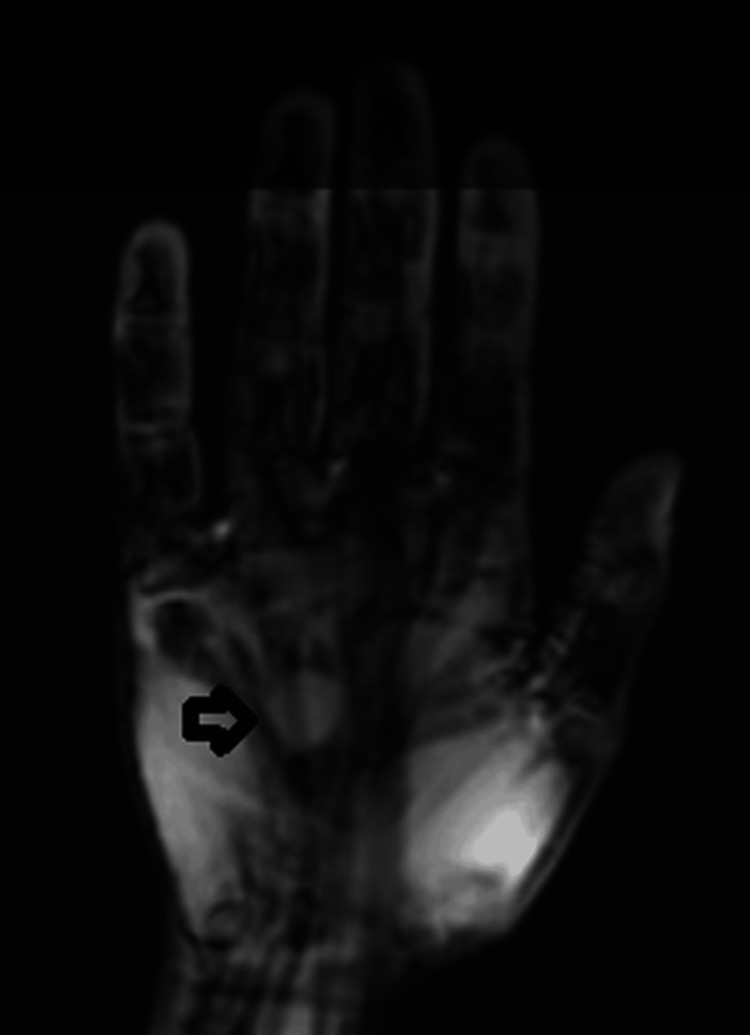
Magnetic resonance imaging of the right hand. MRI of the right hand demonstrating a well-circumscribed ovoid lesion (at arrow) centered at the level of the fourth metacarpal shaft with low signal characteristics on both T1 and T2 sequences and diffuse heterogeneous contrast enhancement.
